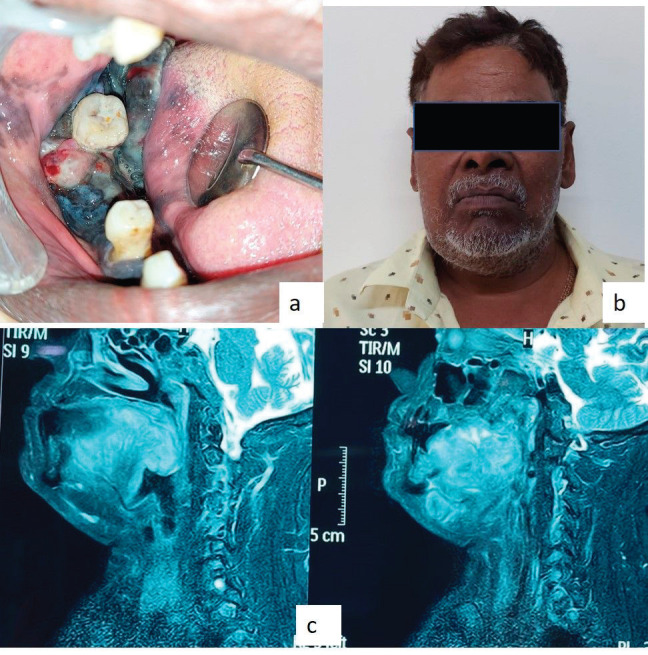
(a–d): Magnetic resonance imaging (MRI) image shows the T2W sagittal section of the lesions.
